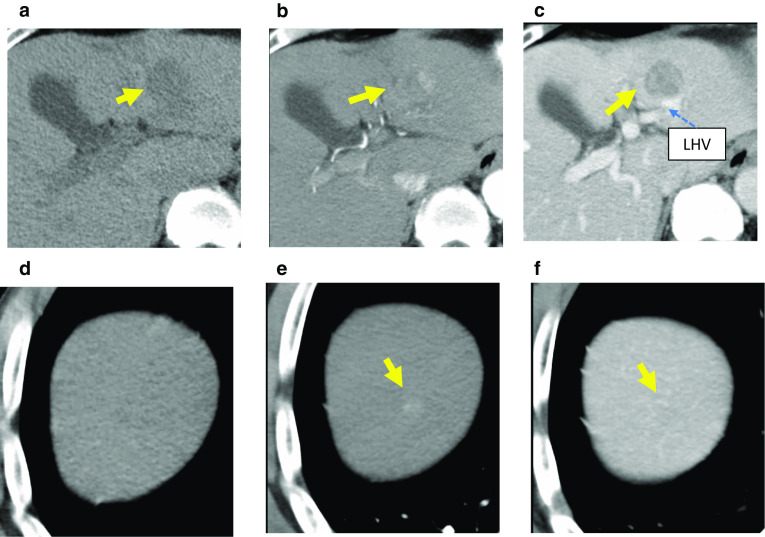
Contrast-enhanced computed tomography (CT) revealed the presence of lesions in the patient’s liver. a A low-density mass, 24 mm in size, in segment III on plain CT. b Enhancement of the lesion in the early phase was noted. c In the late phase, washout was observed. The tumor was in contact with the left hepatic vein (LHV). d The tumor in segment VIII was not clear on plain CT. e An 8-mm tumor was also present in segment VIII of the liver showing contrast enhancement. f Washout was noted in the delayed phase
